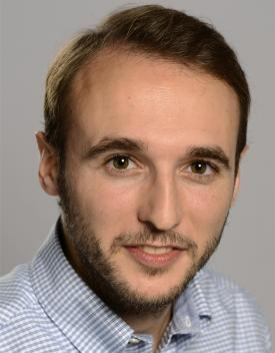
Dr. Federico Fortuni is a cardiologist specialized in cardiovascular imaging. He currently works as a consultant cardiologist at the Cardiology and Intensive Cardiac Care Unit of San Giovanni Battista Hospital in Foligno (Italy). He completed his cardiovascular medicine residency at the University of Pavia and San Matteo Hospital of Pavia. He completed a fellowship in advanced cardiovascular imaging at the Leiden University Medical Center (the Netherlands) and is currently completing a PhD programme in valvular heart disease and advanced cardiovascular imaging at the same university. He was awarded the Young Investigator Award in Clinical Cardiology by the European Society of Cardiology in 2020. He conducted several studies on multimodality cardiovascular imaging with more than 80 articles published in international peer-reviewed journals. He acts as a reviewer for several cardiology journals. He is a member of the Editorial Board of the ‘Journal of the American Society of Echocardiography’, ‘Frontiers in Cardiovascular Medicine’ and ‘Cardiovascular Ultrasound’. He is among the Associate Editors of the ‘European Heart Journal: Imaging Methods and Practice’.
